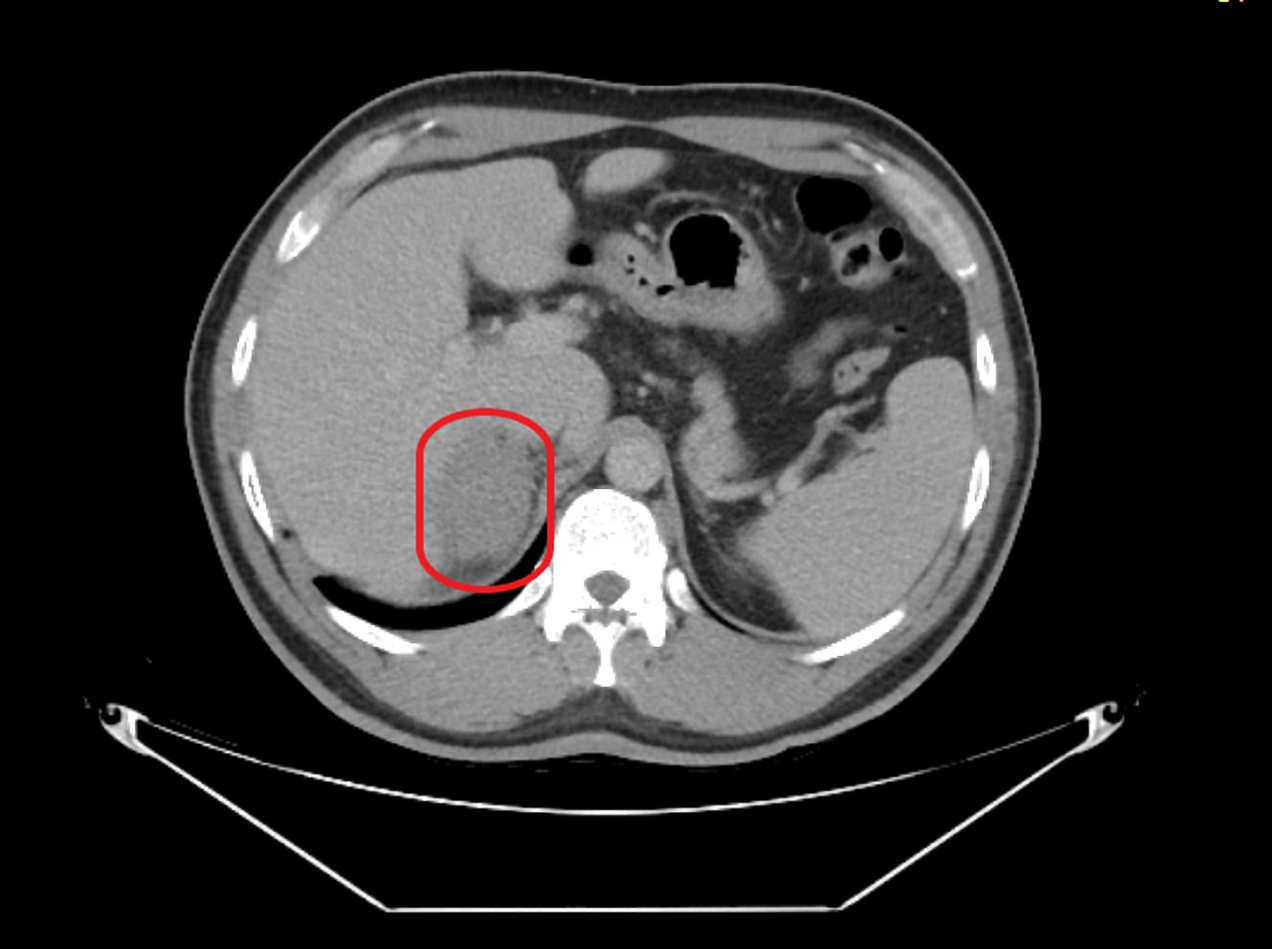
Abdominal computed tomography (CT) scan showing a metastatic lesion in the liver (red circle)
